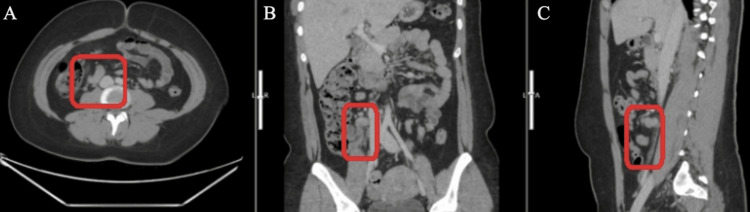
Computed tomography scan of the appendix in the axial (A), coronal (B), and sagittal (C) views The appendix appears thickened and inflamed, dilated up to 16 mm with prominent nearby lymph nodes.
